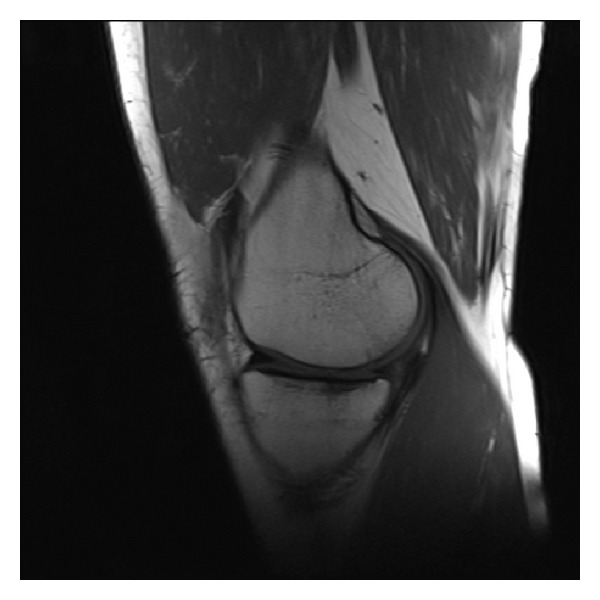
Sagittal MR image demonstrates “absence of the bow tie sign.”
